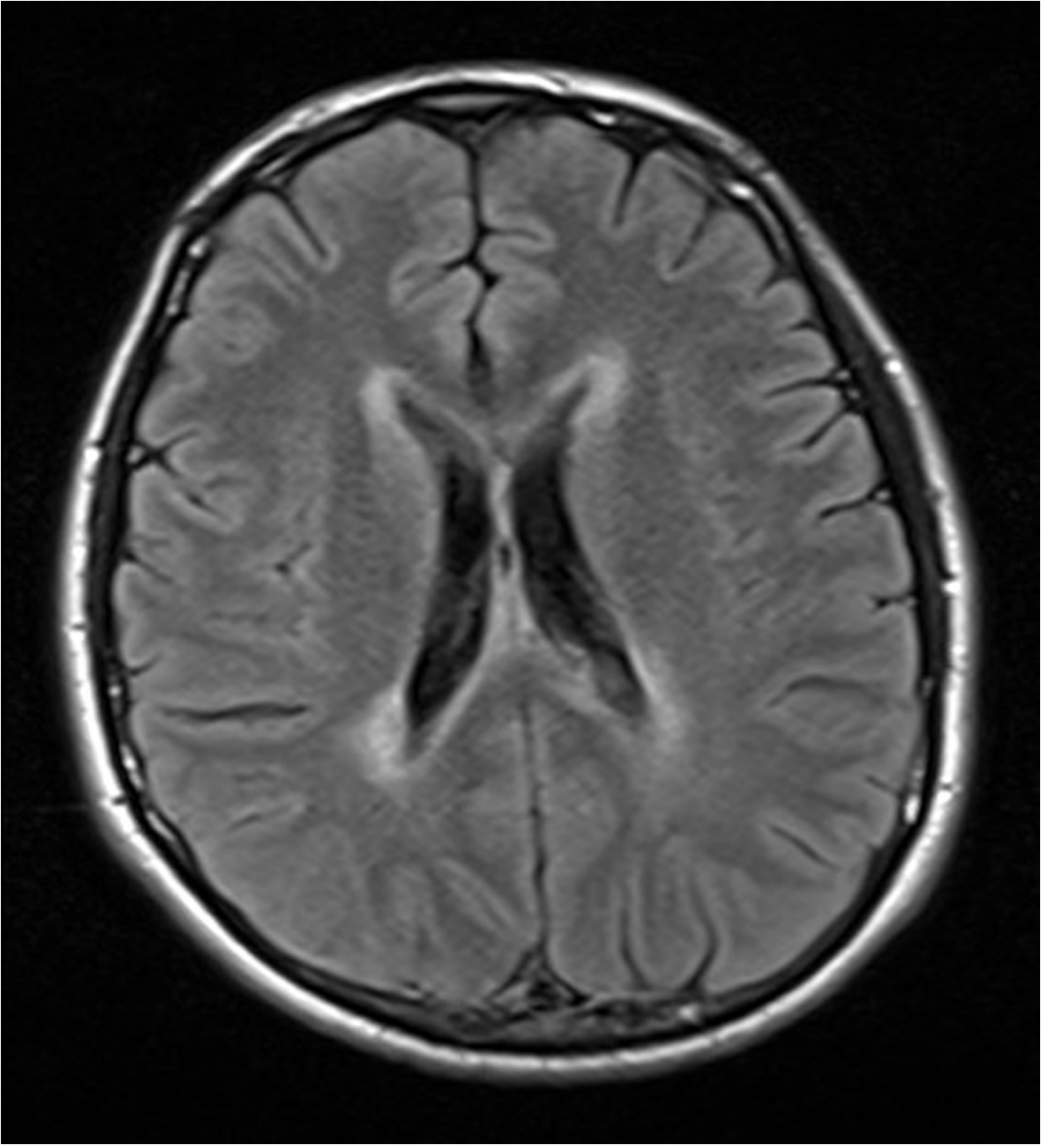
Axial fluid-attenuated inversion recovery magnetic resonance image of the patient shows hyperintense lesions around the ventricles and corpus callosum
